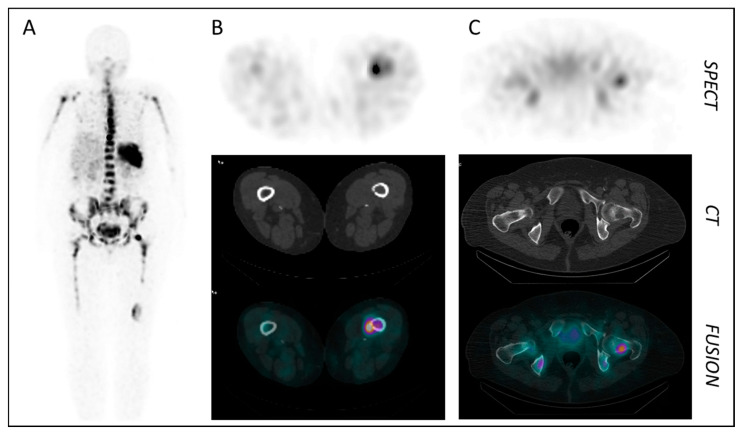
CXCR4-targeted SPECT/CT imaging in a patient with multiple myeloma (MM) using [^99m^Tc]PentixaSPECT. (A) MIP at 3 h p.i. (B,C) Axial SPECT, CT and fusion images of intramedullary MM lesions in the femur (B) and the femoral head (C) (3 h p.i.) [78].
